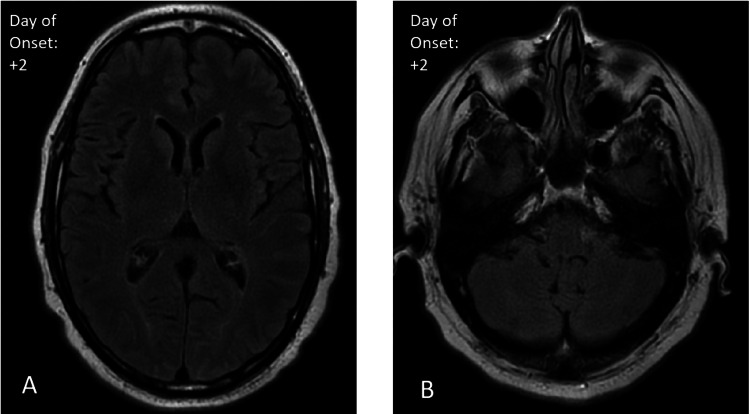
Baseline axial T2 FLAIR MRI. Day two after the onset of symptoms shows normal brain morphology in the cerebrum (A) and the cerebellum (B). There is no evidence of acute ischemia, hemorrhage, edema, or an abnormal mass effect. The brain volume and ventricular system are within normal limits for the patient's age. FLAIR: fluid-attenuated inversion recovery.
